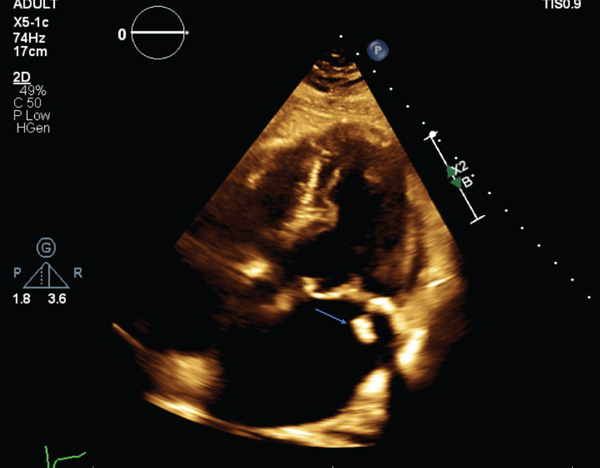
An echocardiogram showed a 1 × 1 cm mobile vegetation on the mitral valve with mild to moderate annular calcification.
